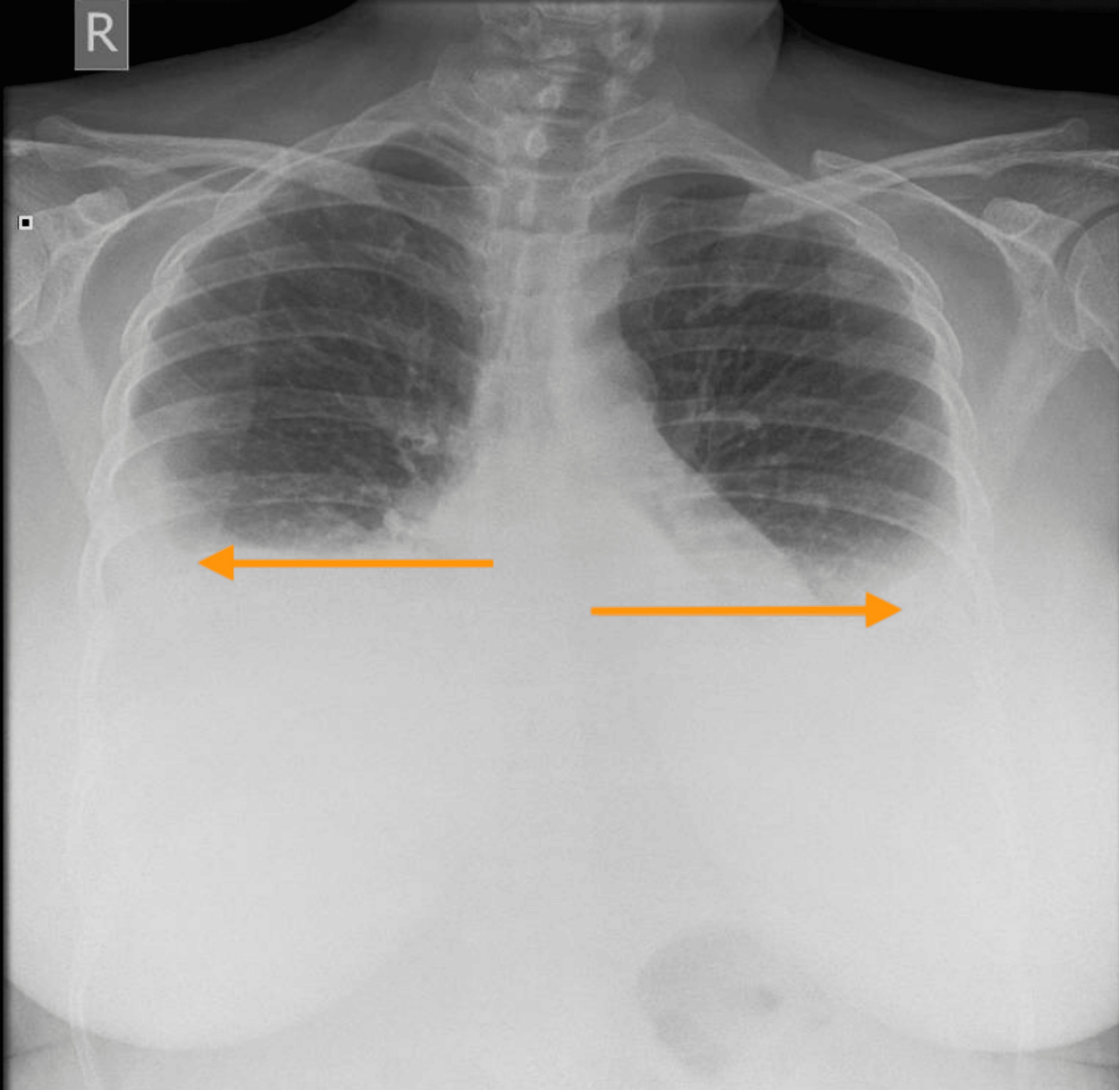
Admission chest X-ray. Bilateral pleural effusions delineated by arrows.
